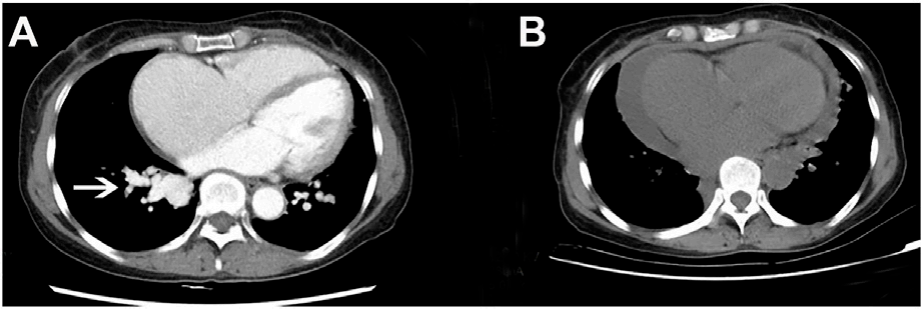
Comparison of chest CT before and after the operation. (A) Chest-enhanced CT showed an enhanced vascular mass in the lower part of the right pulmonary artery (white arrow) before operation. (B) Plain CT scan of the chest revealed that the former vascular mass had disappeared after the operation.
